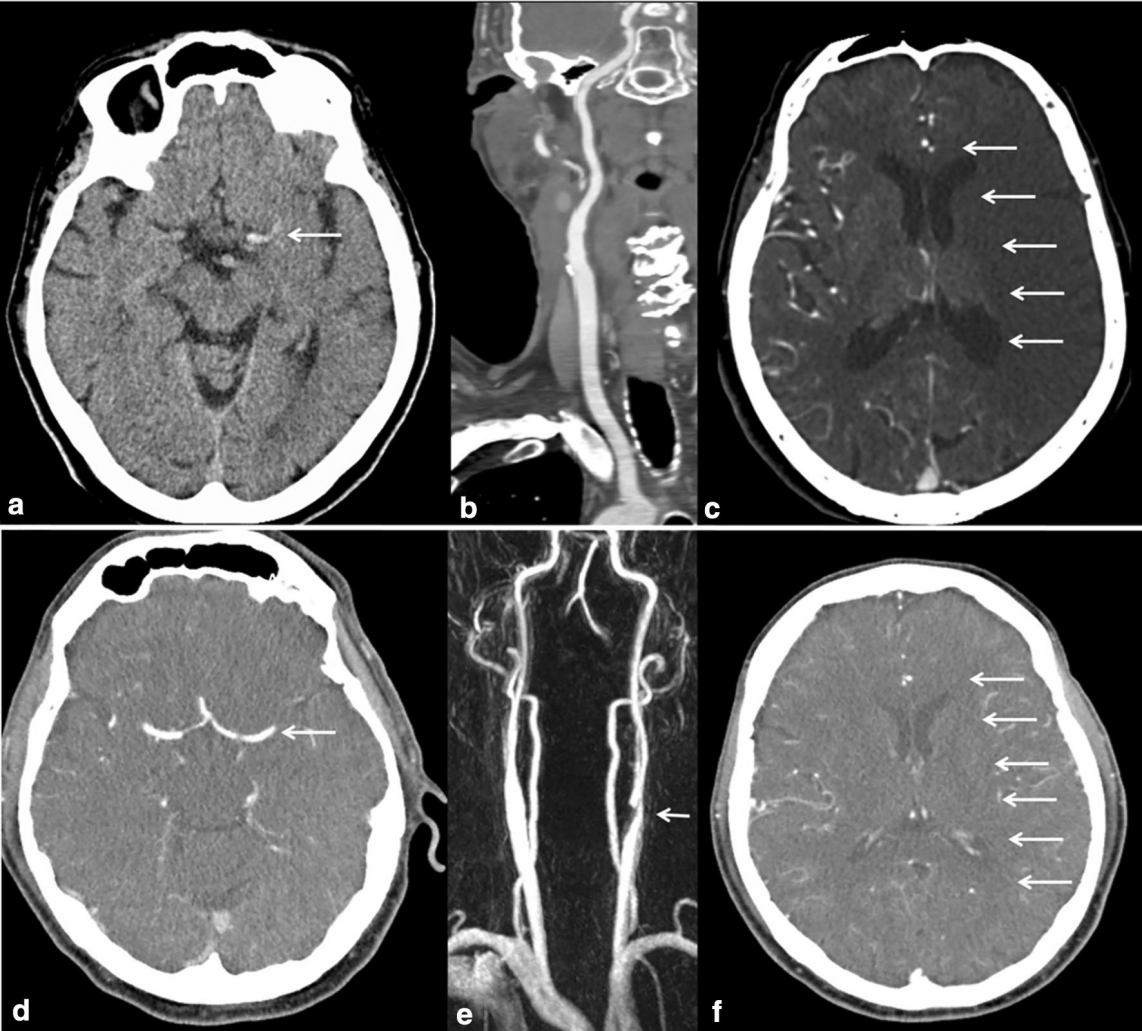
Exemplary cases of acute LVO stroke patients with good and poor collaterals. a Shows left sided hyperdense media sign (arrow) on preinterventional CT scan of a patient with acute occlusion of the M1 segment of the middle cerebral artery without an indication of ipsilateral carotid artery stenosis (b). Intracranial CT angiography (CTA) displays unfavorable collateral status according to a Tan collateral score of 0 before thrombectomy (c, arrows). Another patient presents with acute left sided atheroembolic M1 occlusion (d, arrow) and high-grade extracranial carotid artery stenosis on preinterventional CTA, which is demonstrated in e (arrow). f shows favorable collaterals according to a Tan collateral score of 3 (arrows)
